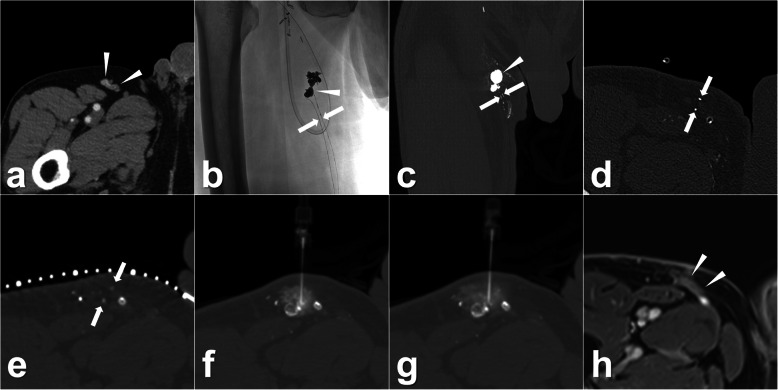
Images from a patient who underwent a melanoma resection 1 year before. a Contrast-enhanced CT showed a suspicious metastatic lymph node (white arrowheads) at the right proximal thigh. Hence, a right inguinal lymphadenectomy was performed, and the postoperative pathological result confirmed the metastasis of the lymph node. Because of the postoperative LL, the therapeutic TL was performed showing definite iodinated oil extravasation (white arrowhead) from two lymphatic vessels (white arrows) using both fluoroscopy (b) and a CT scan, obtaining coronal (c) and axial (d) reconstructions. Twenty-eight days after TL, percutaneous ALVS was performed owing to the continuous leakage. e The two target afferent lymphatic vessels (white arrows) close to the leakage could still be identified. f During the puncture, the needle tip was located between the two afferent lymphatic vessels, and 1 mL of contrast fluid was injected that showed an ideal distribution covering both the target lymphatic vessels. g After injection of 2.0 mL of 95% ethanol, a CT scan was performed which showed a nebulous dispersal of the contrast completely covering the two target lymphatic vessels. One day later, the leakage ceased and the drainage could be removed. The patient was discharged on the same day. h Around 3 months later, magnetic resonance imaging showed no tumour recurrence and fluid accumulation. A mild enhancement (white arrowheads) at the region of sclerotherapy could be observed which indicated clinically inapparent scarring after ALVS. In the further follow-up for 1 year, no relapse of the tumour or lymphatic leakage was found. *ALVS* Afferent lymphatic vessel sclerotherapy, *CT* Computed tomography, *LL* Lymphatic leakage, *TL* Transpedal lymphangiography
